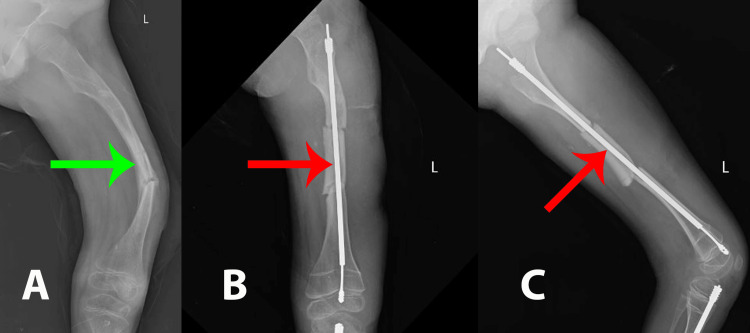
(A) Preoperative X-ray with severe bowing of the left femur with significant curvature (indicated by the green arrow), a characteristic deformity seen in osteogenesis imperfecta. The bone appears thin and fragile, with a notable varus deformity. (B) Immediate postoperative X-ray demonstrating the placement of an intramedullary rod in the left femur indicated by the red arrow. There is also evidence of osteotomy, with proper alignment of the femoral segments. (C) Another immediate postoperative lateral view X-ray with the intramedullary rod in place along the left femur. The red arrow indicates the position of the rod, confirming the corrected alignment from a different perspective. This lateral view highlights the successful stabilization and positioning of the rod, further ensuring the bone's structural support post surgery.
